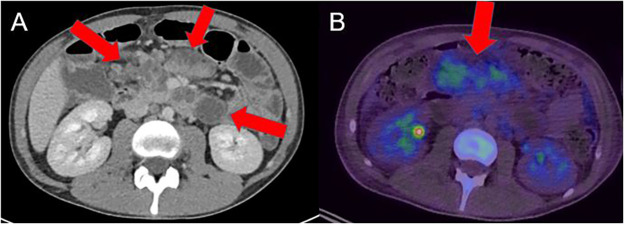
A, Contrast-enhanced abdominal CT showing conglomerated lymph nodes consistent with bulky disease. B, PET/CT demonstrating FDG uptake in multiple mesenteric lymph nodes in the abdominal region. CT, computed tomography; FDG, fluorodeoxyglucose; PET, positron emission tomography.
